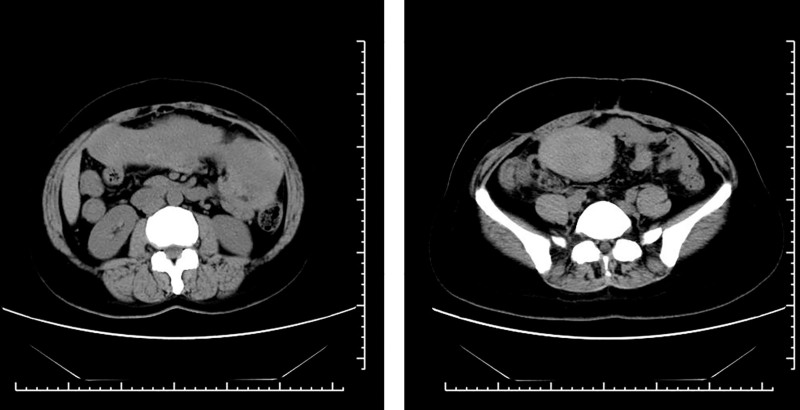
Case 1 patient’s follow-up CT image 5 years post-diagnosis (managed with active surveillance). cDNA = complementary deoxyribonucleic acid, CT = computed tomography.
